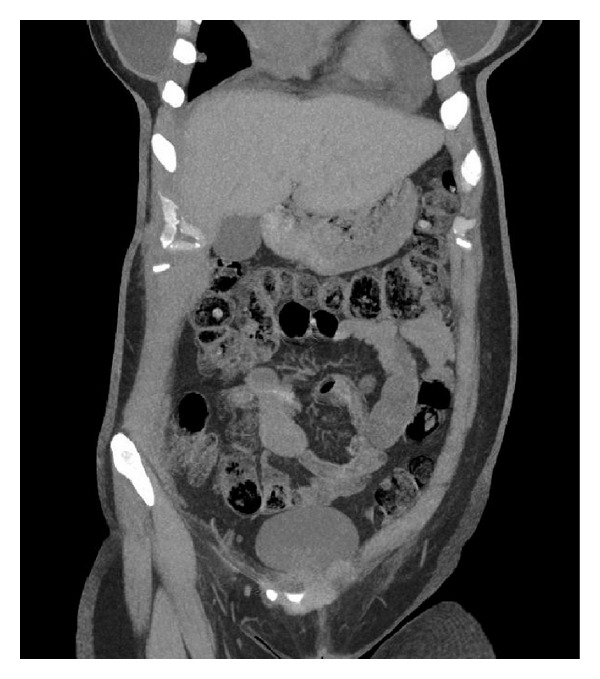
Abdominal CT scan with evidence of small bowel intussusception.
